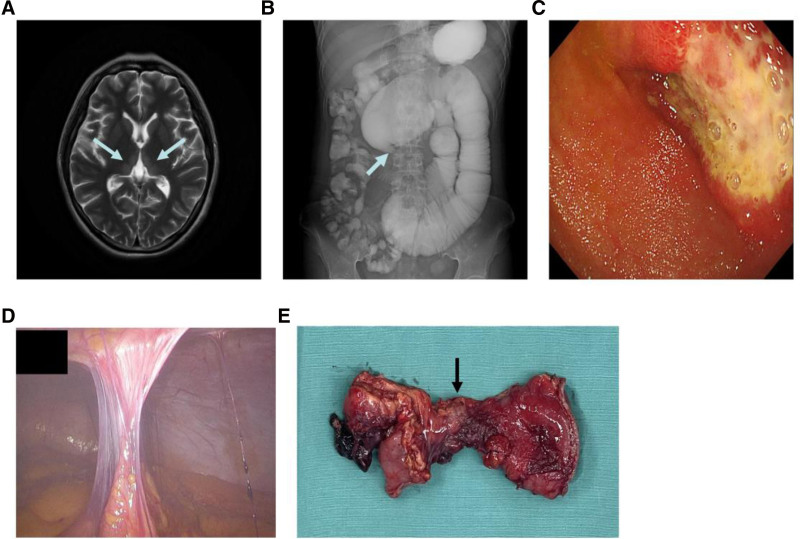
(A) Hyperintense signal shadows are seen near the third ventricle, bilateral thalamus and midbrain ducts (blue arrow), considering Wernicke encephalopathy. (B) Gastrointestinal radiography revealed local stenosis of the jejunum with beak-like changes (blue arrow) and obvious dilatation of the proximal jejunum. (C) Circumferential jejunal stenosis with ulceration. (D) Adhesion was found between mesentery, omentum, and anterior abdominal wall. (E) A 9 cm of the jejunum was resected, the stenotic segment (black arrow) was 2 cm in length.
